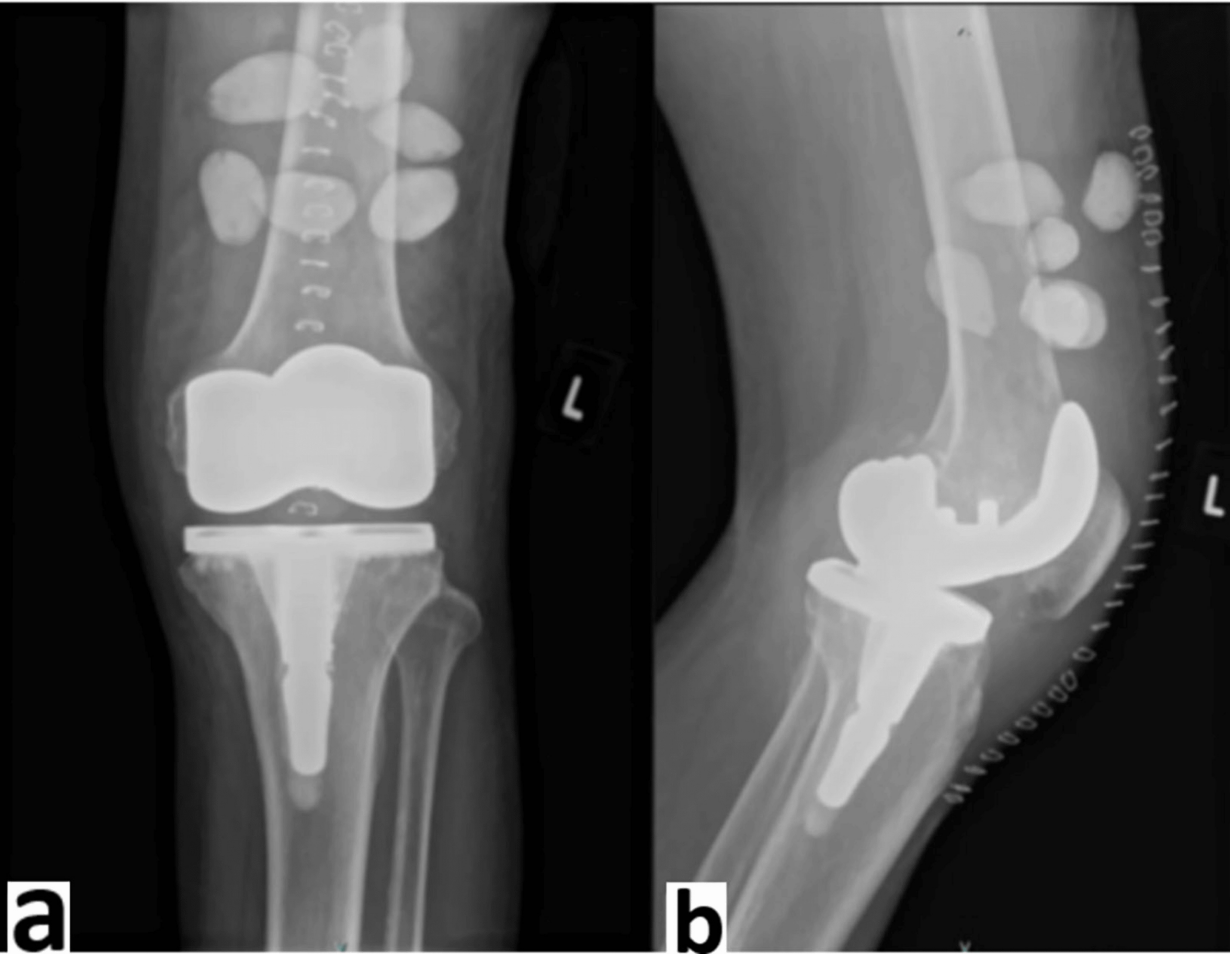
Radiographs of left knee post insertion of vancomycin beads a) anteroposterior view; b) lateral view
